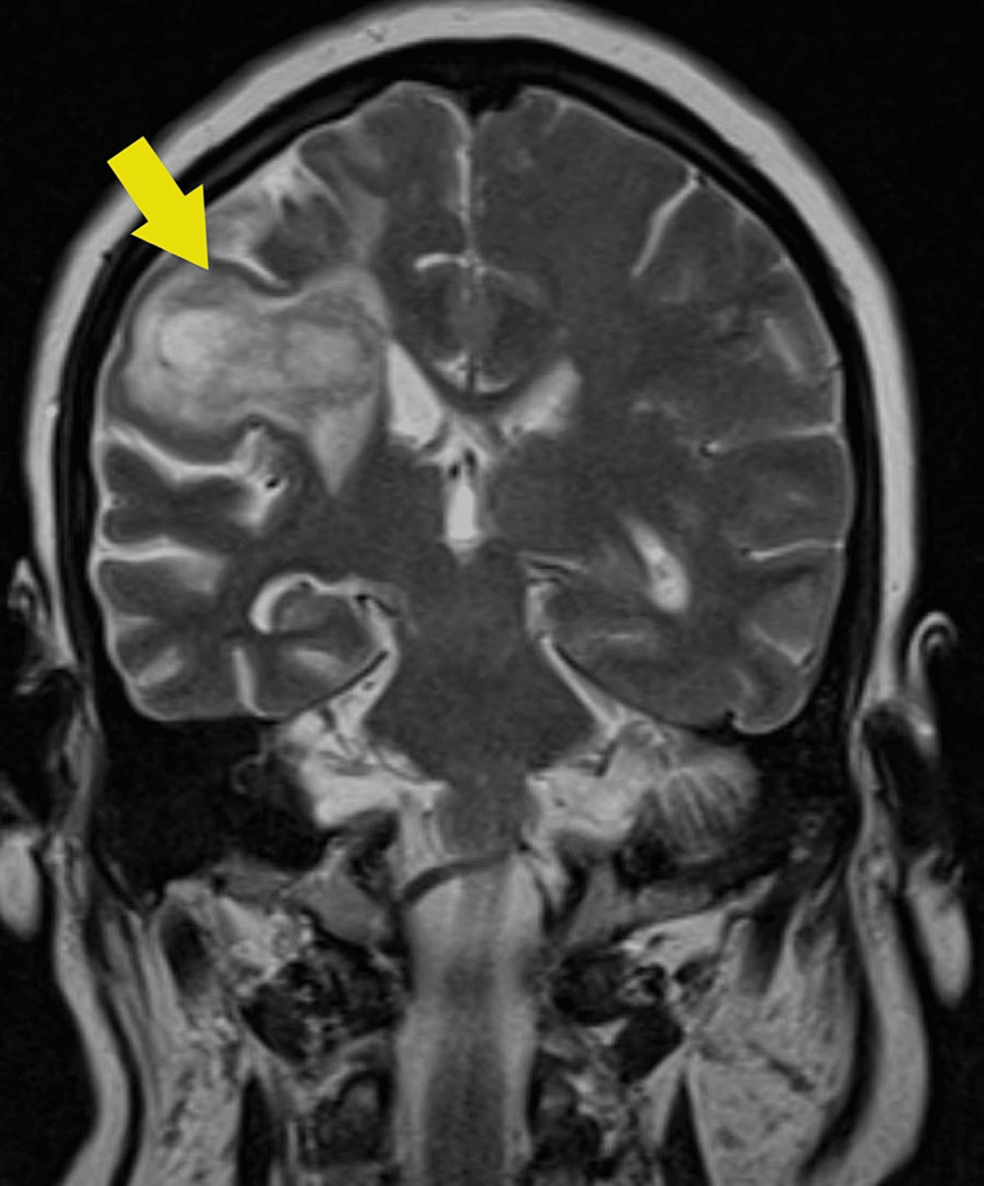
MRI brain coronal view. The yellow arrow indicates the location of the abscess
